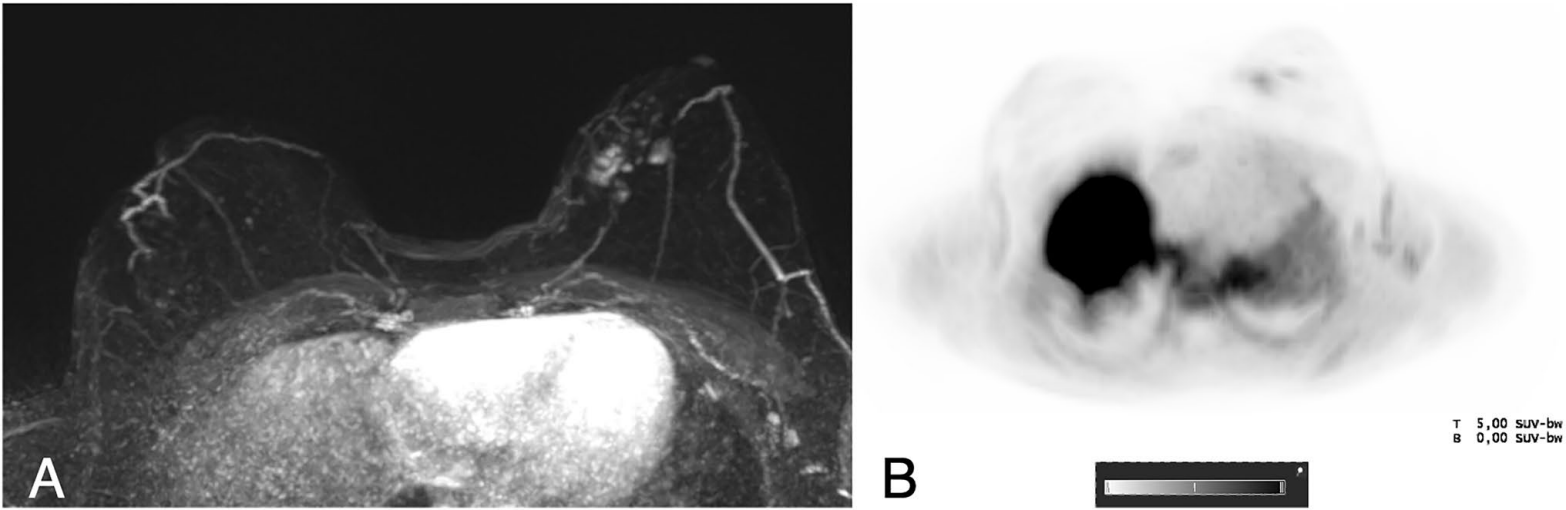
A 51-year-old woman with a suspicious palpable finding in the left breast. Contrast-enhanced MRI revealed a multifocal tumor in the medial quadrants of the left breast (a, T1 post-contrast subtracted images, Maximum Intensity Projection (MIP), white circle). Lymph nodes were slightly larger in the left axilla, but with no suspicious morphology (white arrow). The lesion showed a weak 18F-Fluoroethylcholine uptake (SUV_max_ tumor 1.74, PET MIP images in b, black circle), as well as a weak uptake by the lymph nodes. Surgery revealed a multifocal invasive carcinoma non-special type grade 2, luminal A with a low proliferation rate and no lymph node metastasis
